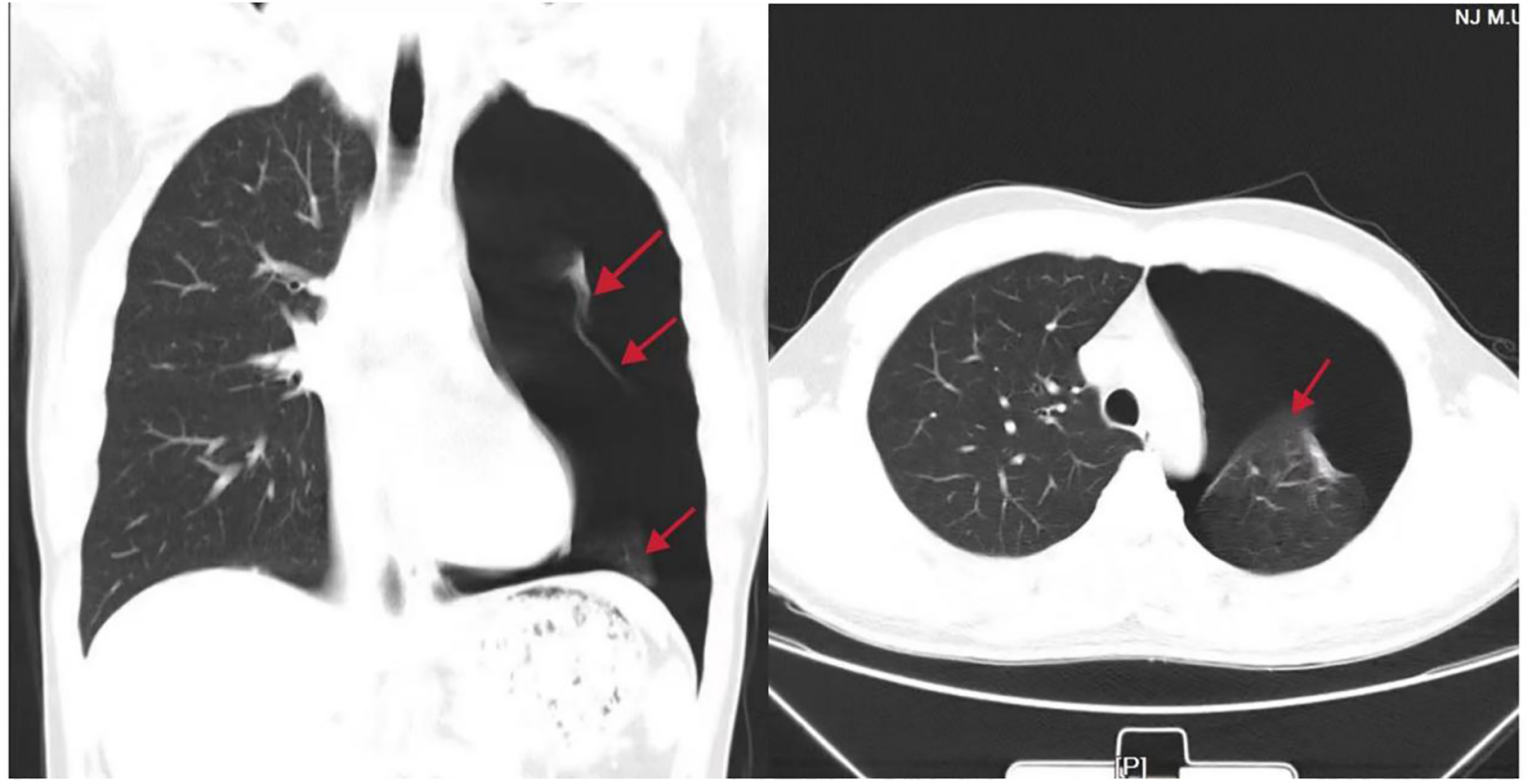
Preoperative chest radiograph and computed tomography scan showing an 80%–90% left-sided pneumothorax (red arrow).
